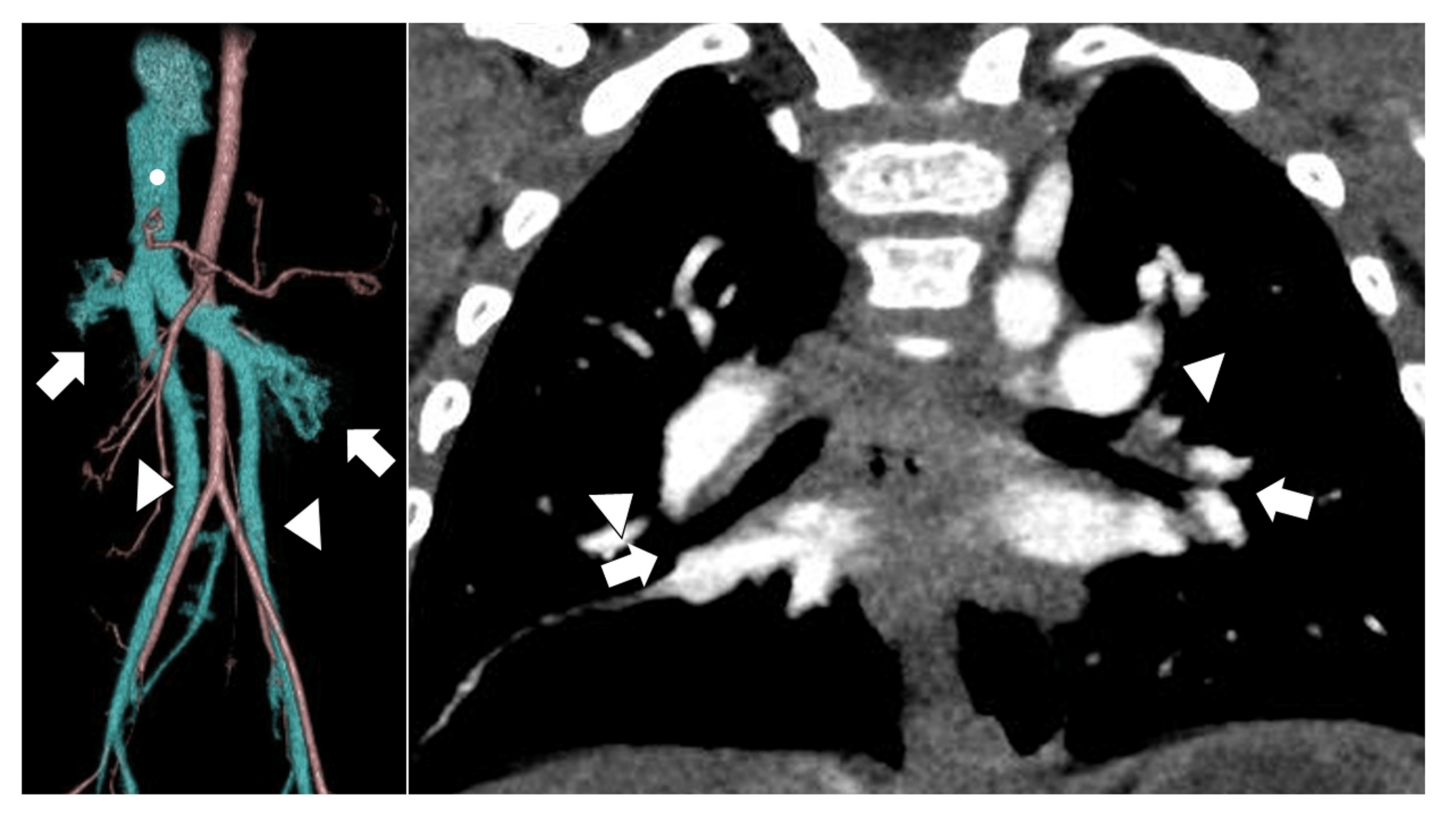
Vascular structures on CT A: The left and right renal veins (arrow) drain separately into the left and right iliac veins (arrowhead). The iliac veins subsequently converge to form a single IVC (circle). B: The CT image was obtained at the time of intracardiac repair. It shows that both main bronchi (arrow) pass below their corresponding pulmonary arteries (arrowhead). CT: computed tomography; IVC: inferior vena cava
